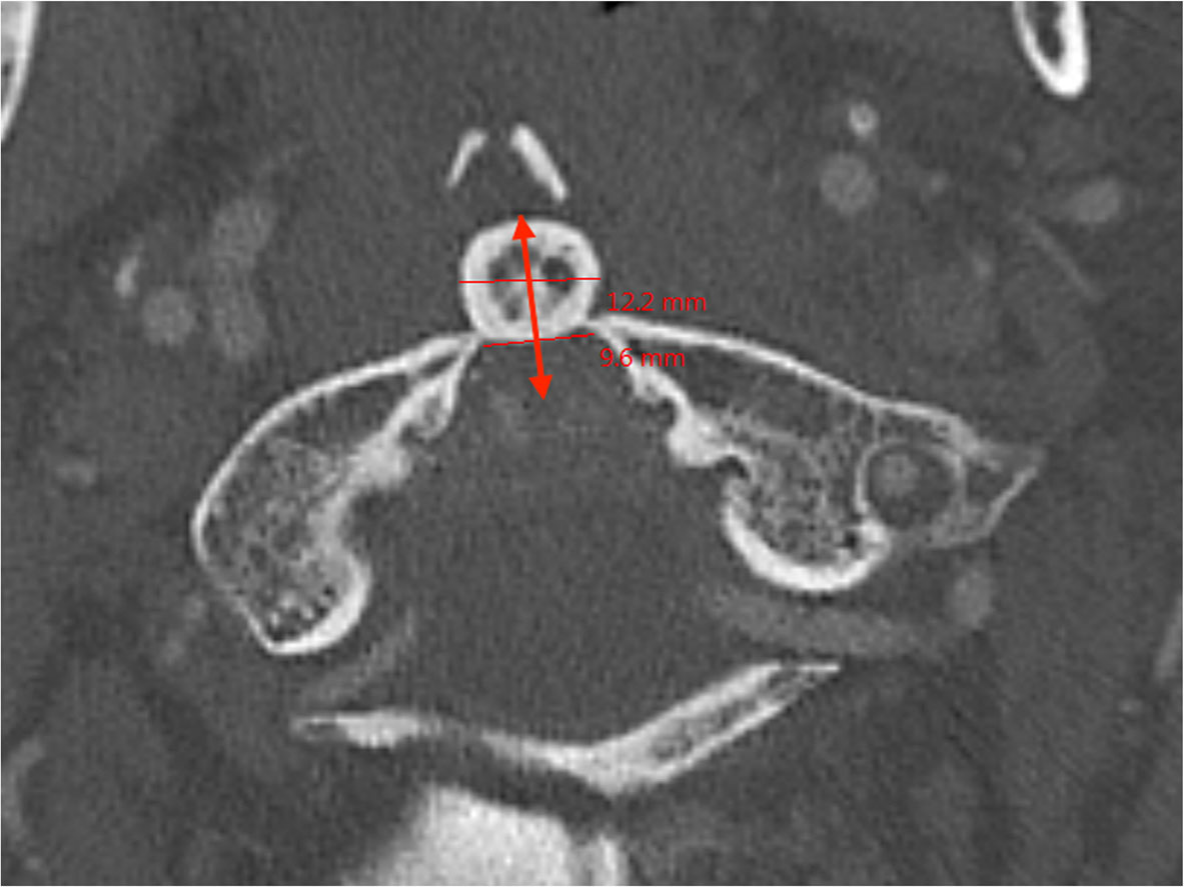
Axial slice of pre-reduction CT showing that the distance between the two fragments of the anterior arch of C1 less than the diameter of the odontoid process. The arrow displays the negative ADI from the anterior aspect of the dens to the posterior aspect of the anterior arch of C1
